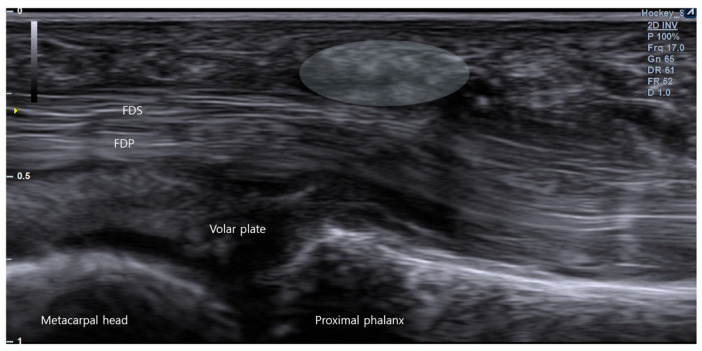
Ultrasonographic demonstration of A1 pulley thickening in trigger finger. A longitudinal sonographic view over the volar aspect of the metacarpophalangeal (MCP) joint. Pathological thickening of the A1 pulley is visualized as a hypoechoic bulge (demarcated by the white shaded area) overlying the flexor digitorum superficialis (FDS) and profundus (FDP) tendons.
